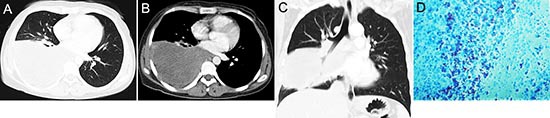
pPNET in a 30-year-old woman CT imaging showed an irregular iso-dense mass in the right lower lung (A) that invaded the mediastinum (B). Coronal images using lung windows demonstrated invasion of the right lower lobe bronchus (C). The poorly differentiated small round tumor cells were CD99 positive (D × 100).
